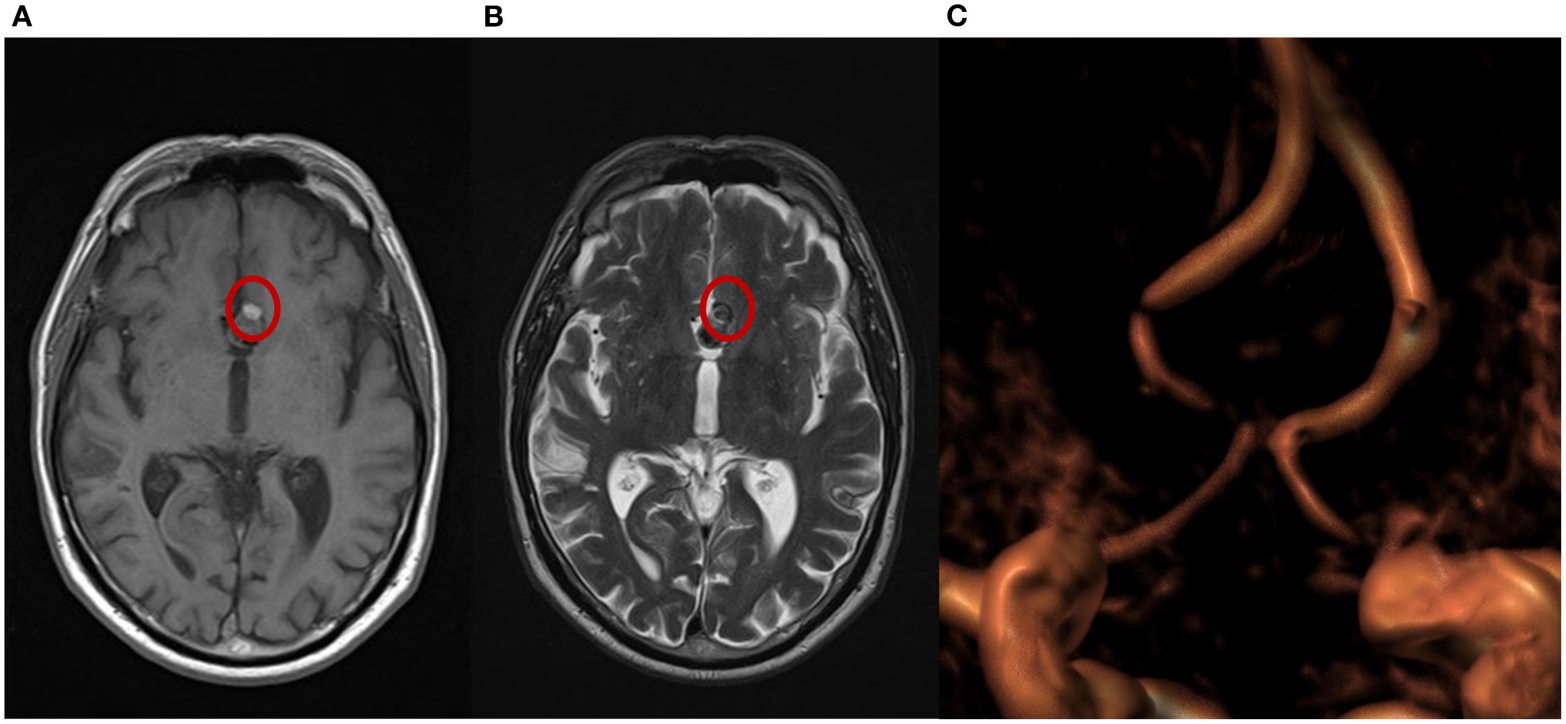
Magnetic resonance imaging (MRI) from a patient with complete occlusion at 3-month follow-up. (A) T1 weighted spin echo (T1W SE); a small portion of hyperintensity can be visualized at the bottom of the aneurysm. (B) T2 weighted turbo spin echo (T2W TSE); a small portion of isointensity in the same area shows a different grade of cloth maturation in the thrombosis process occurring in the sac after the treatment. (C) A 3-dimensional time of flight (TOF 3D) volume rendering image shows no flow in the aneurysm.
